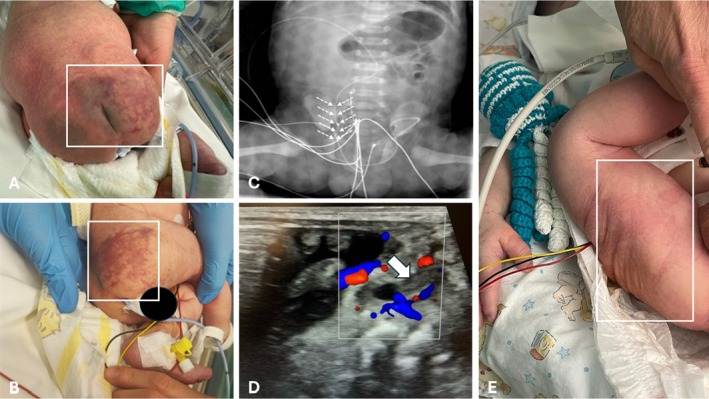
Violaceous macular skin lesions on the right buttock and loin, panels A and B (white boxes). X‐ray showing misplacement of the UAC in the right internal iliac artery, panel C (white dashed arrows). Doppler ultrasound of the right internal iliac artery showing a clot in the vessel lumen and absence of blood flow, panel D (white arrow). Resolution of the cutaneous lesions with near complete restitution to baseline after 48 h of treatment with unfractionated heparin and topical therapy, panel E (white box).
